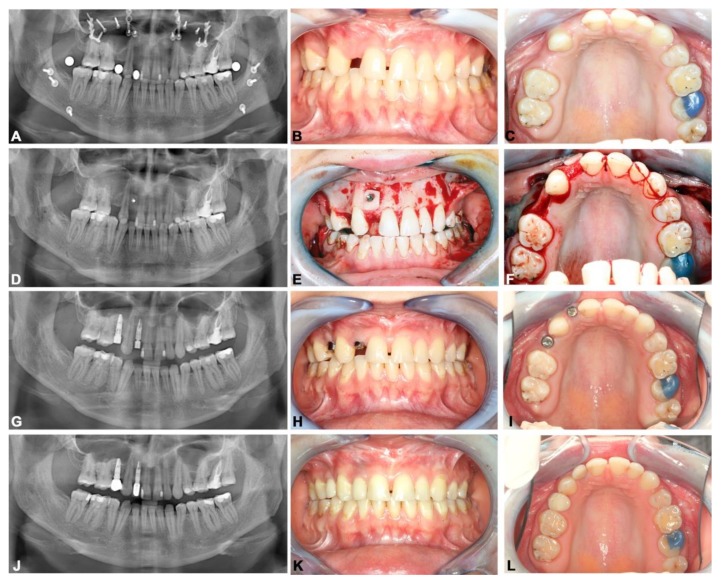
Dental rehabilitation of patient with hypodontia. (A–C) Panoramic radiograph and intraoral photos showing the situation after orthognathic surgery and teeth gaps in the left maxillary: first premolar and lateral incisor region. (D–F) Panoramic radiograph and intra-operative photos showing bone augmentation in the left maxillary lateral incisor region. (G–I) Panoramic radiograph and intraoral photos after the exposure of the implants. (J–L) Panoramic radiograph and intraoral photos after 54 months of prosthetic rehabilitation.
